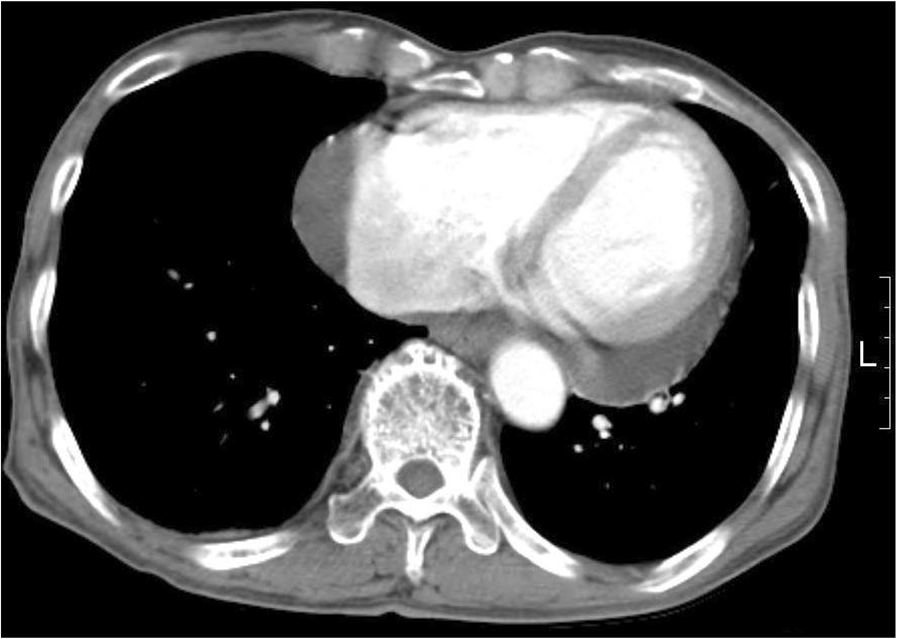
Preoperative computed tomography scan revealed pericardial effusion.
